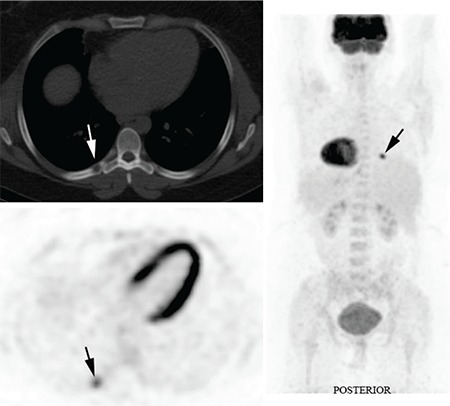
The PET study revealed a solitary focal intense FDG uptake inthe right 7th rib posteriorly (solid black arrow). The concurrent low dose CTscan of the Philips Gemini 64 PET/CT camera confirmed the presence of awell-defined lytic lesion with partial bony erosion at the site of the intenselyincreased FDG uptake (solid white arrow)
